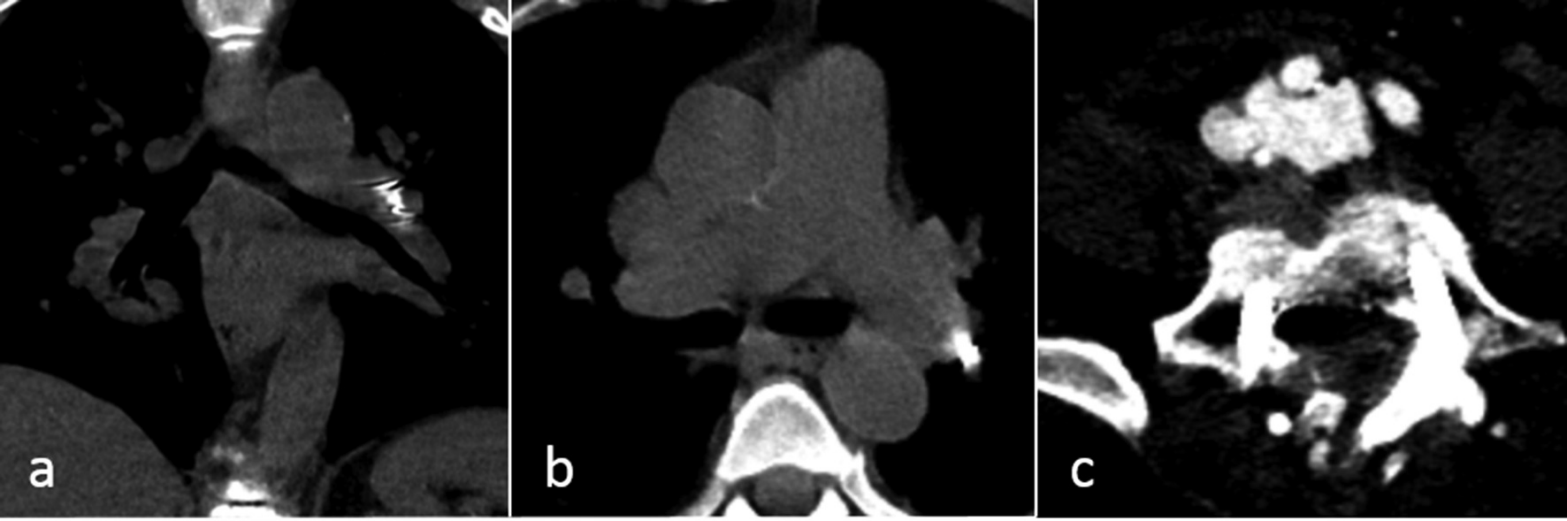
CT scans on the 2nd postoperative day. (a, b) show coronal and transversal non-contrast-enhanced CT images of the chest. The transforaminal lumbar interbody fusion spacer (arrows) has embolized to the left pulmonary artery, partly extending into the lower lobe artery. Contrast-enhanced image (c) of the prior transforaminal lumbar interbody fusion location proves its embolization. The arteriovenous fistula originating from the right common iliac artery is now more clearly identifiable.
